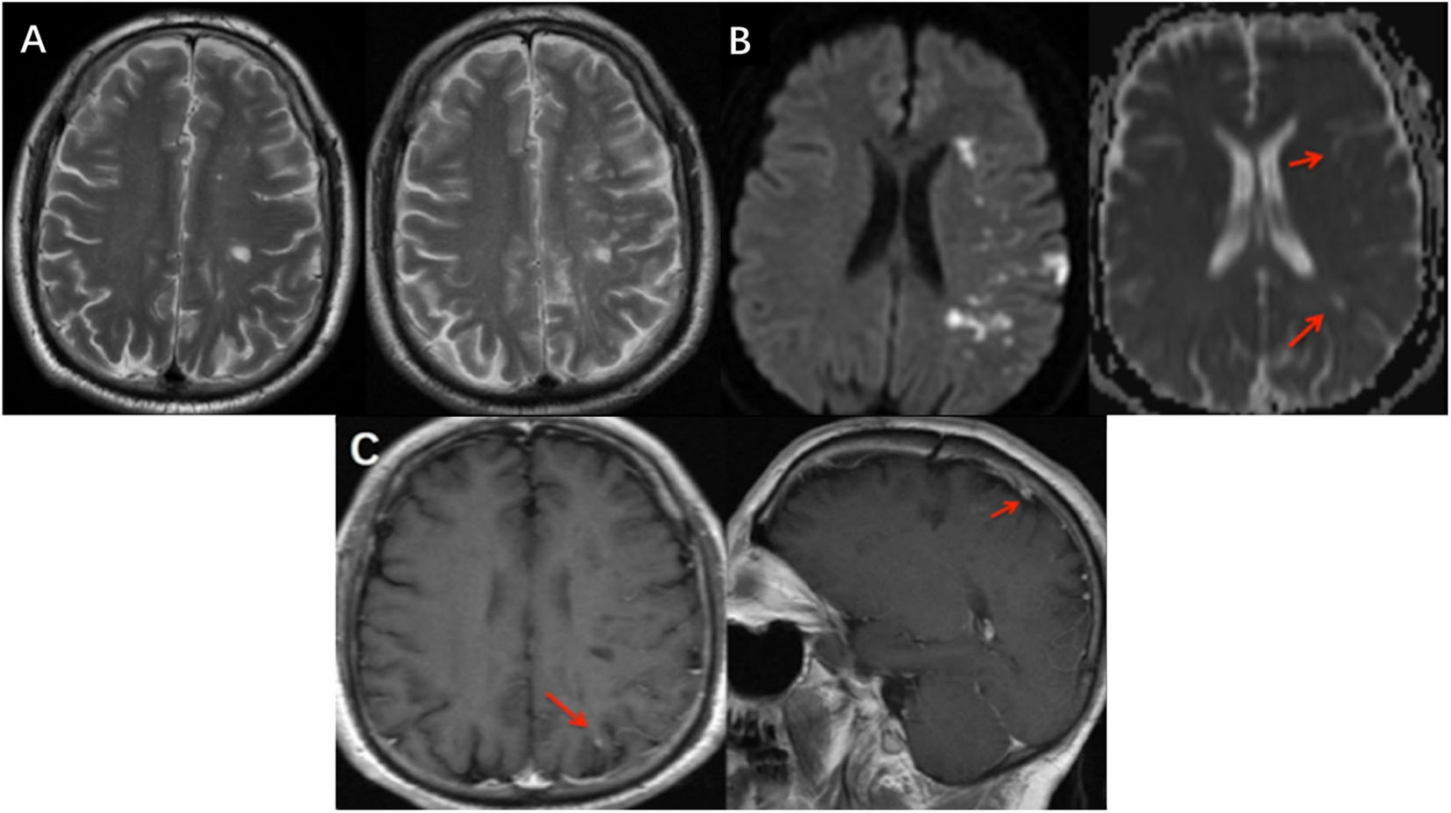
Imaging features at different MRI scans. (A) New T2 lesions on EMRI (A-right) compared to the first MRI (A-left) which demonstrated DIT. (B) EMRI scan showed hyperintensity in the left frontal–parietal lobe and lateral ventricle nearby in DWI; however, these lesions were both hyperintensity and hypointensity signals in ADC. Red arrows showed hypointensity lesions. (C) Cranial EMRI scans revealed that lesion with an enhanced phase signal in the left frontal–parietal lobe (C-left), and also seed enhanced phase lesion in sagittal view (C-right). EMRI, emergency cranial MRI; DIT, dissemination of lesions in time; MRA, magnetic resonance angiography; ACA, anterior cerebral arteries; MCA, middle cerebral arteries; PCA, posterior cerebral artery.
